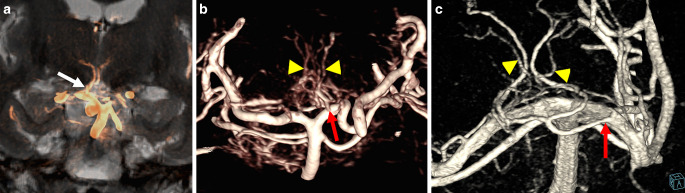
Artery of Percheron. a coronal maximum intensity projection (MIP) of the artery of Percheron (arrow) projected onto coronal T2 weighted MR image. b posterior thalamoperforating arteries (PTA, arrowheads) emanating from a netlike unilateral structure (arrow). c volume rendering of the basilar tip and the P1-segments showing the artery of Percheron (arrow) and accessory small penetrating branches (arrowheads) originating from a netlike structure surrounding the basilar tip and the right P1-segment
